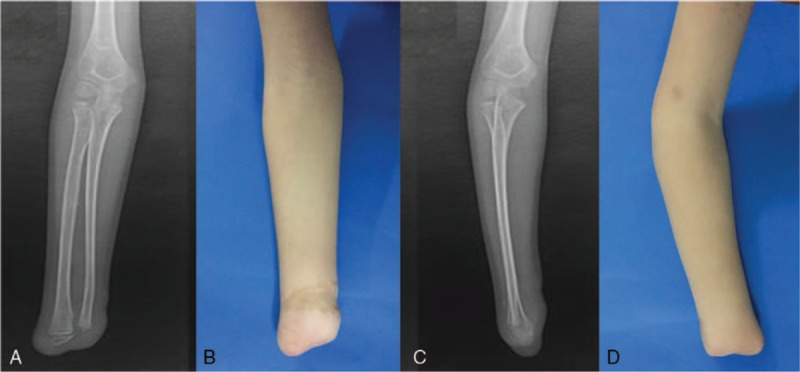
Radiographic and macroscopic examinations (anteroposterior: A, B; lateral: C, D) of the affected limb after a wrist disarticulation of the 8-year-old child.
